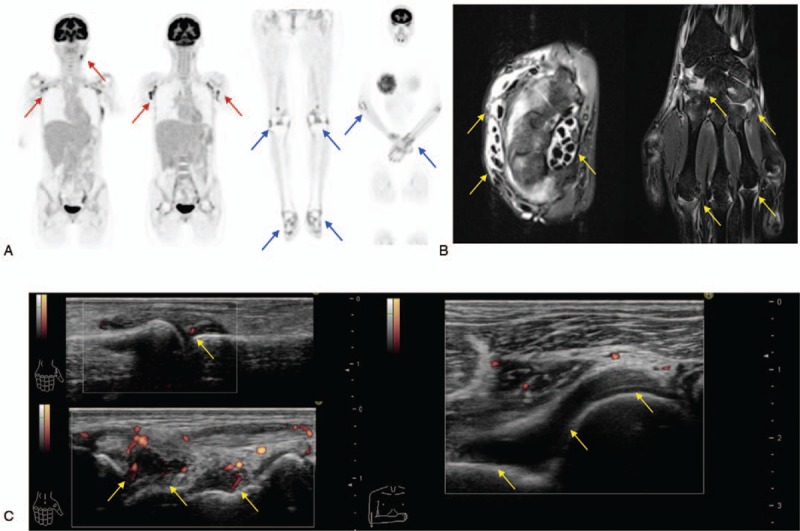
(A) Fluoro-2-deoxy-d-glucose accumulation visible in her left cervical lymph node (red arrows); axillary lymph nodes (red arrows); as well as elbow wrist, knee, and ankle joints (blue arrows). (B) Magnetic resonance imaging shows active synovitis and tenosynovitis in her right hand (yellow arrows). (C) Musculoskeletal ultrasonography assessment in the 1st metacarpophalangeal joint and carpal joints show synovial thickening with high-intensity power Doppler signals (yellow arrows). Synovial thickening is present in the humeroradial joint (yellow arrows).
